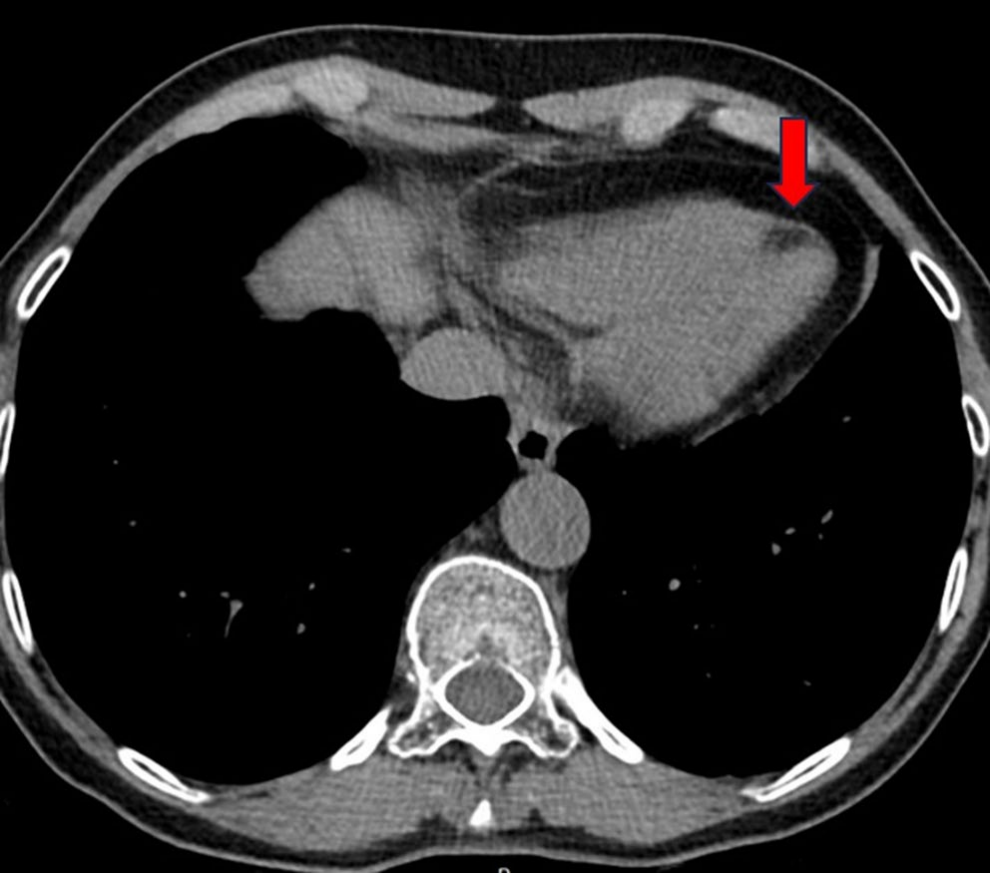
Thoracic CT scan showing a hypodense lesion with negative attenuation values (-77 Hounsfield units) located at the apex of the left ventricle (red arrow)
